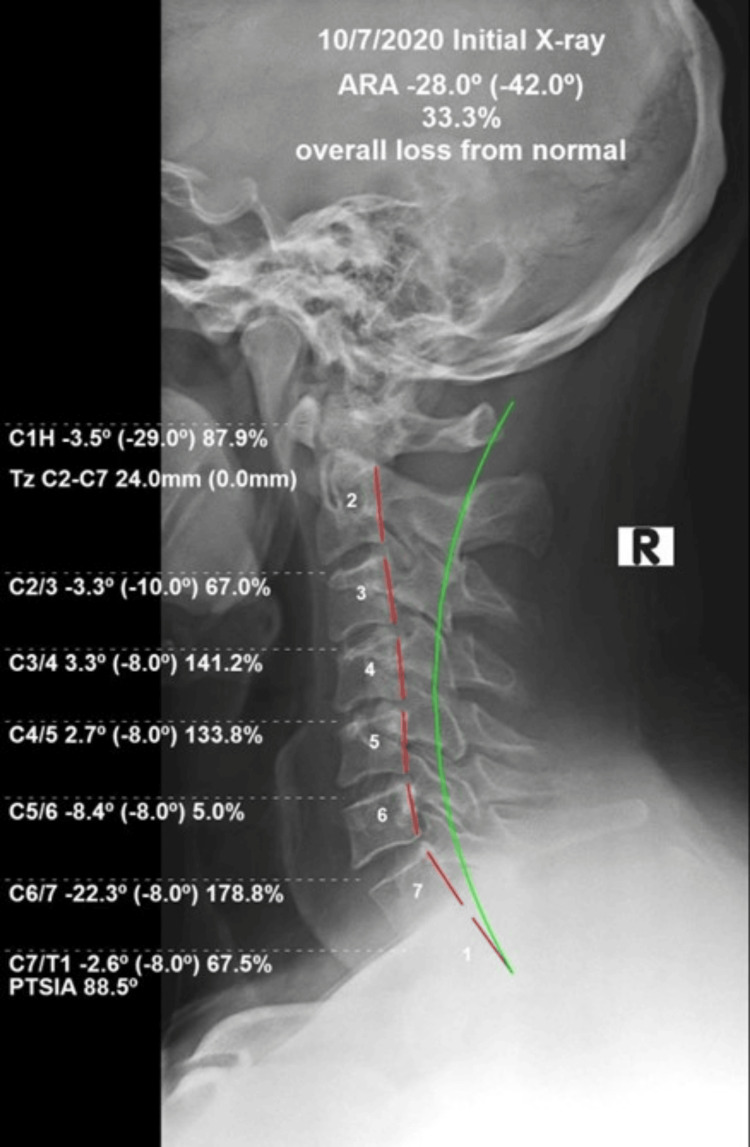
Post-MVC pre-treatment lateral cervical radiograph The red dashed line indicates the abnormal position of the vertebrae using the Harrison Posterior Tangent Method. The green line represents ideal cervical lordosis.
